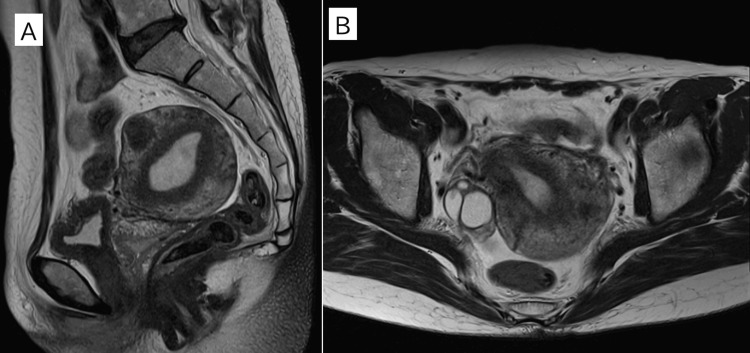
Preoperative magnetic resonance imaging findings. (A) Sagittal T2-weighted magnetic resonance image demonstrating an enlarged uterus with multiple intramural leiomyomas showing heterogeneous low-to-intermediate signal intensity. (B) Axial T2-weighted magnetic resonance image showing multiple uterine leiomyomas and a mildly thickened endometrium. MRI: magnetic resonance imaging
